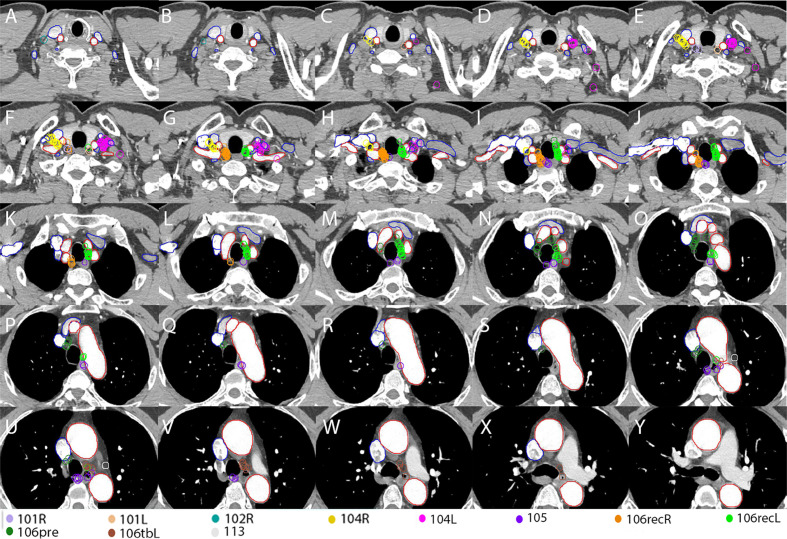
Continuous cross-sectional images (a thickness of 5.00 mm) with all of the TVnds on the template CT (A–Y). Red lines: arteries; Blue lines: veins.
